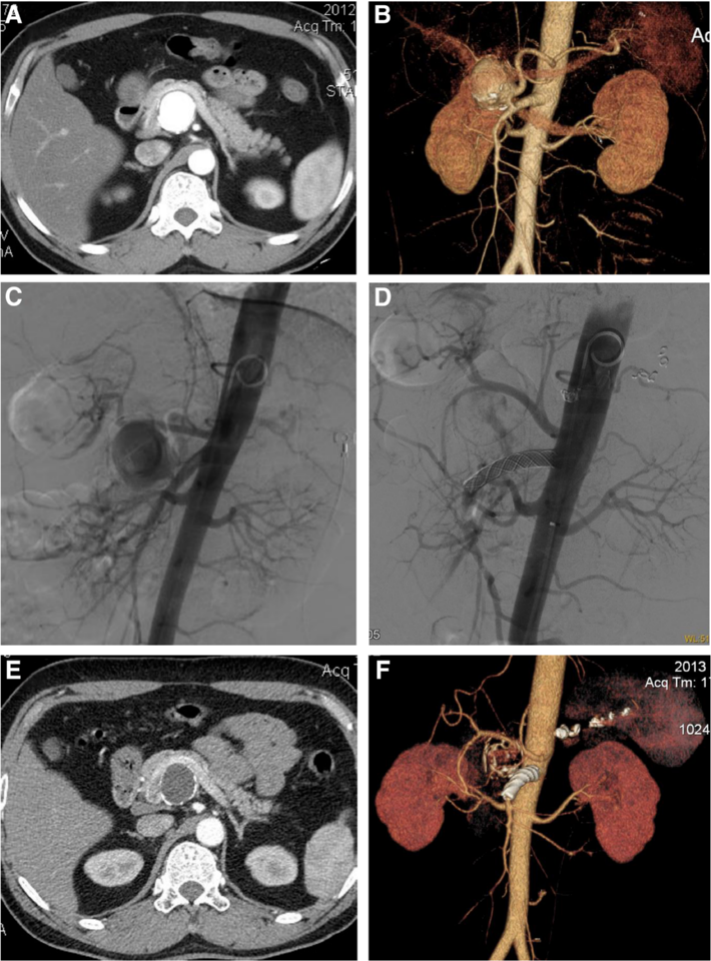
The image finding of case one during preoperation, intraoperation and follow-up. A and B, Preoperative computed tomographic angiography (CTA) confirmed a 40 mm × 38 mm splenic artery aneurysm (SAA) near the origin of the superior mesenteric artery (SMA). C, Intraoperative digital subtraction angiogram (DSA) demonstrated the findings same as CTA. D, The completion aortogram revealed disappearance of the aberrant SAA lumen and patency of the SMA but a tiny endoleak. E and F, Follow-up abdominal CT scan in 12 months postoperatively confirmed an occluded aneurysm sac with marked shrinkage and patency of the SMA.
